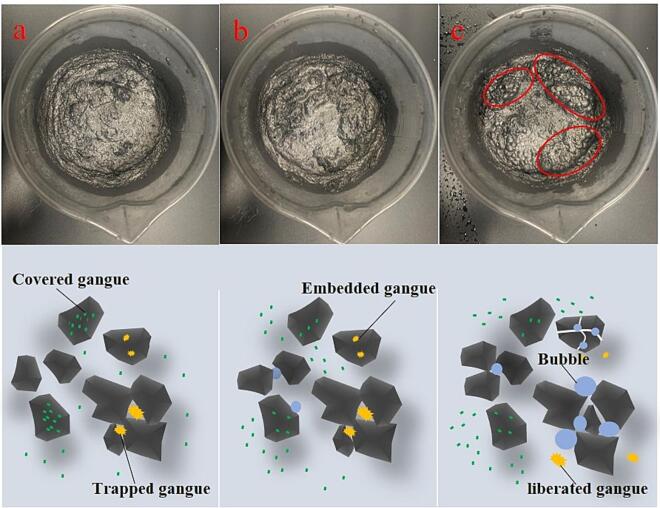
Graphite slurry after pretreated by different methods (a: without pretreatment; b: bath-type ultrasound with intensity of 100%, 4 min; c: horn-type ultrasound with intensity of 100%, 1 min).
